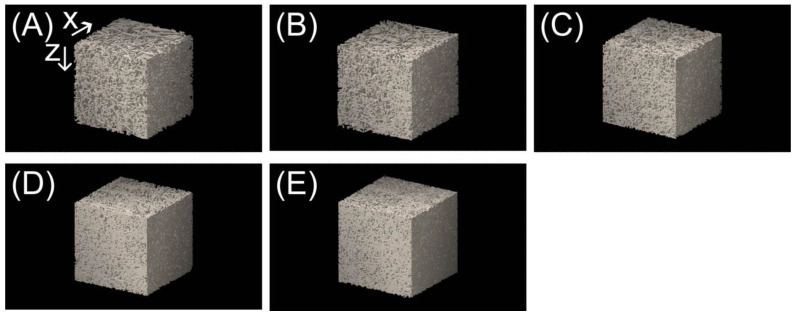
X-ray computed tomography images. The sample thickness in the *z* direction is 0.500 mm; the width in the *x* and *y* directions is 0.503 mm. (A) 8-ply laminated product, (B) 10-ply laminated product, (C) 12-ply laminated product, (D) 14-ply laminated product, (E) 16-ply laminated product.
